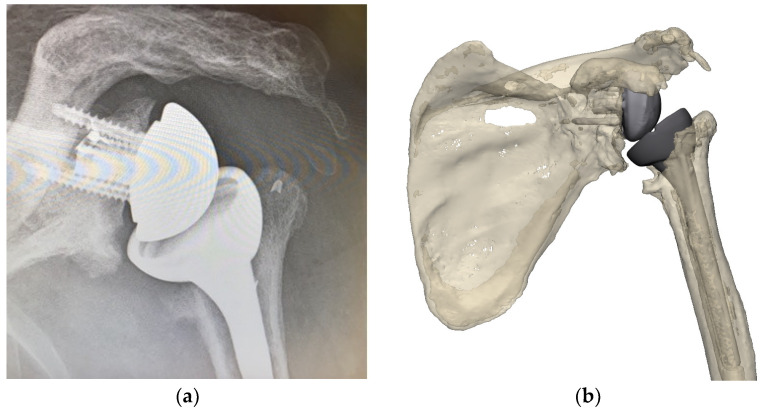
Preoperative X-ray of glenohumeral joint (a) and 3D reconstruction showing malpositioning of primary implants in situ (image from Reflect COMPLEX^TM^ (Akunah, Brisbane, Australia)) (b).
